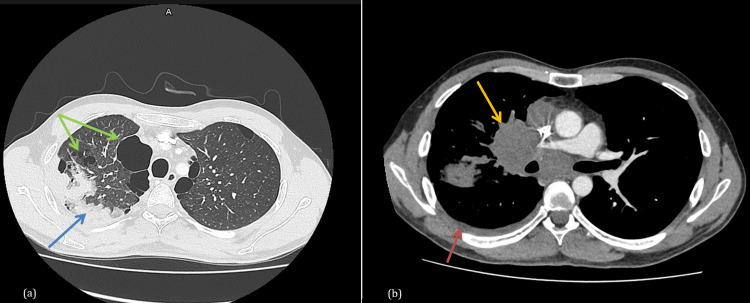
Computed tomography (CT) of the chest (a) Lung window showing: Multiple confluent intraparenchymal masses of the right upper lobe (blue arrow) associated with emphysema, bullous dystrophy, and diffuse bronchial syndrome (green arrows). (b) Mediastinal window showing voluminous mediastinal lymph nodes (yellow arrow), and pleural effusion on the right side (red arrow).
